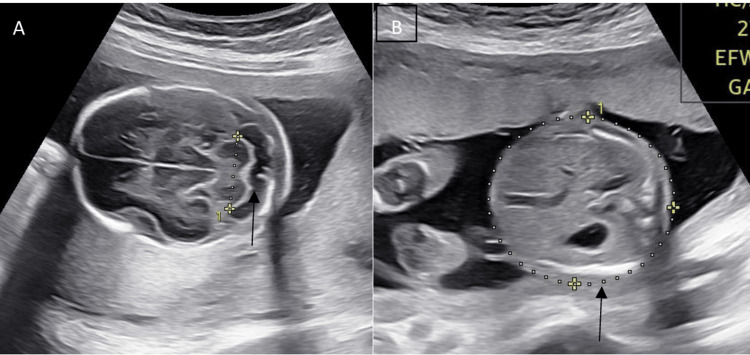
Measurement of ultrasound parameters Ultrasonographic measurement of (A) TCD (black arrow) and (B) AC (black arrow) TCD: transcerebellar diameter; AC: abdominal circumference
